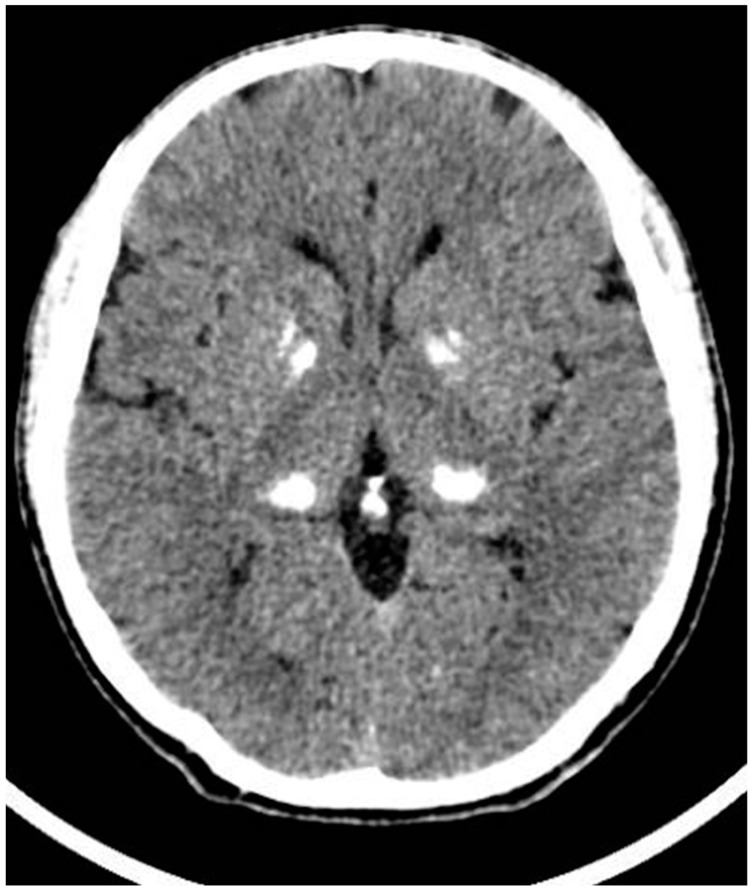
Fahr syndrome. CT scan showing bilateral calcifications of the basal ganglia, particularly present in the dorsal thalamus, globus pallidus, and putamen areas.
